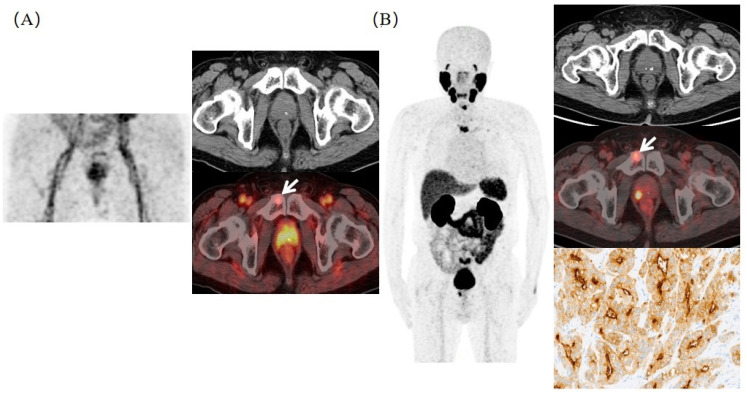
Typical ^68^Ga-PSMA-11 PET/CT images in detecting osseous metastasis. (A) The right pubic bone metastasis (white arrow) was visible at 3 min P.I. (SUV_max_ = 5.1). (B) Corresponding bone metastasis (white arrow) also displayed at 45 min P.I. (SUV_max_ = 8.2), which was confirmed in pathological results.
